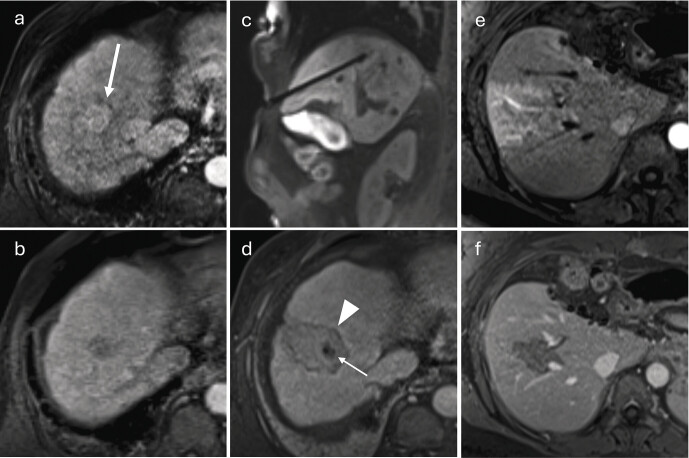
MRI-guided microwave ablation.
